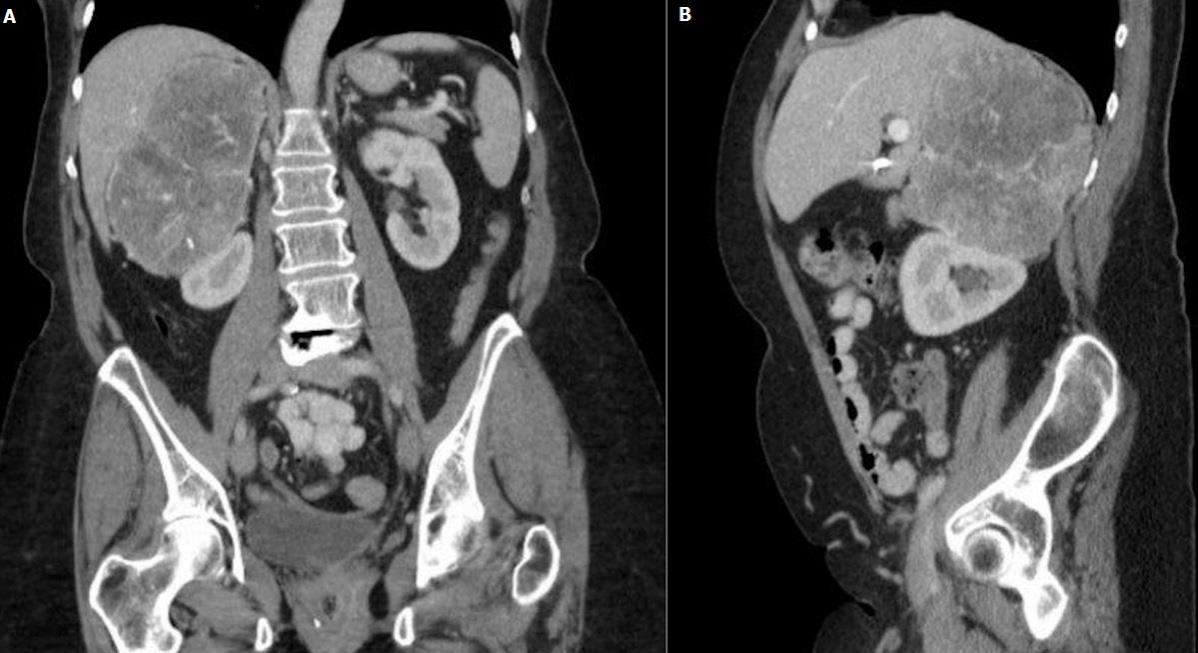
CT abdomen: (A) coronal section showing 8cm mass above the right kidney; (B) sagittal section showing the adrenal tumor
